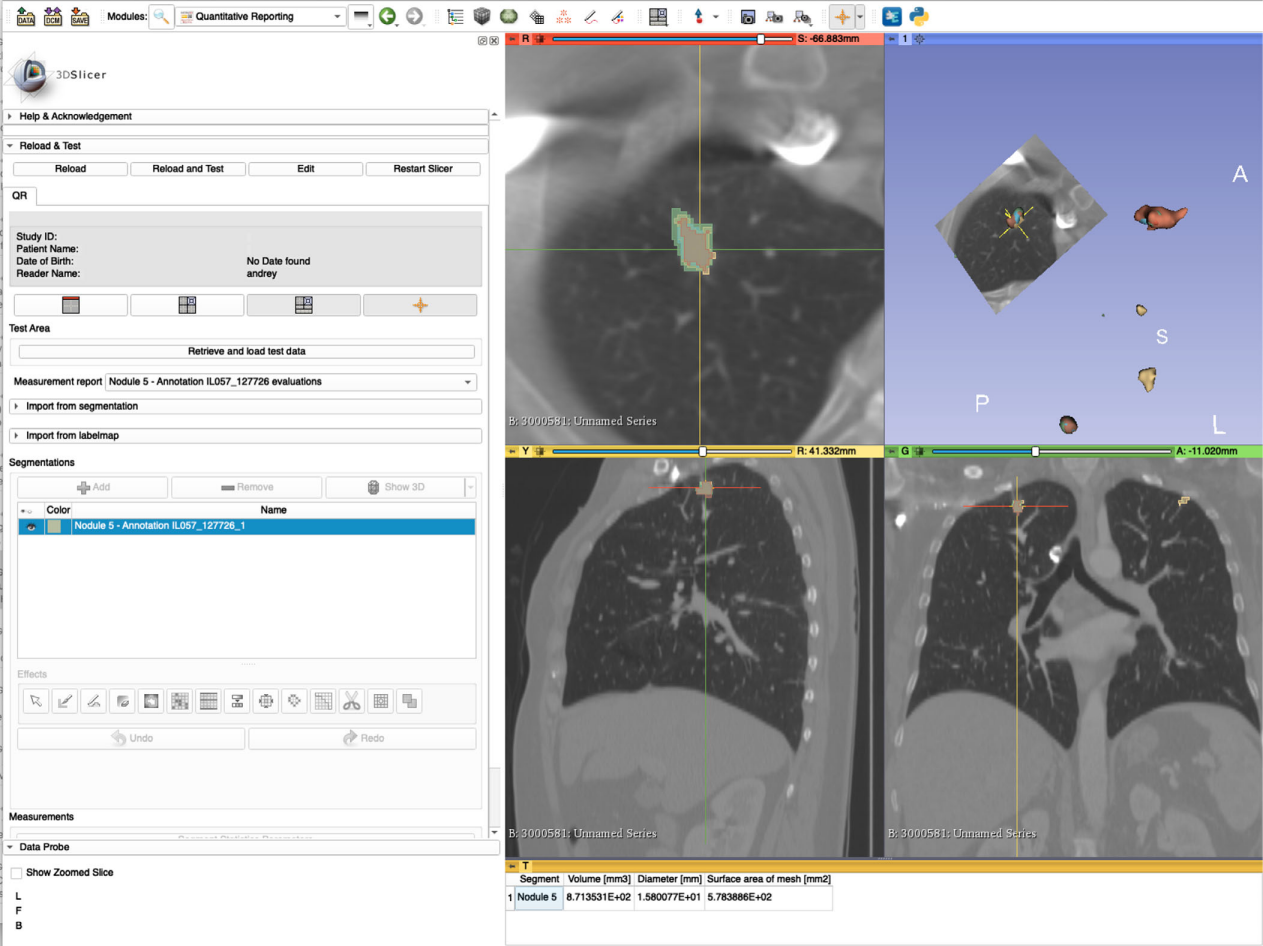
Example of visualization of the annotations and the associated measurements using 3D Slicer QuantitativeReporting extension. Shown is one of the CT scans and the corresponding annotations for subject LIDC‐IDRI‐0055. [Color figure can be viewed at wileyonlinelibrary.com]
